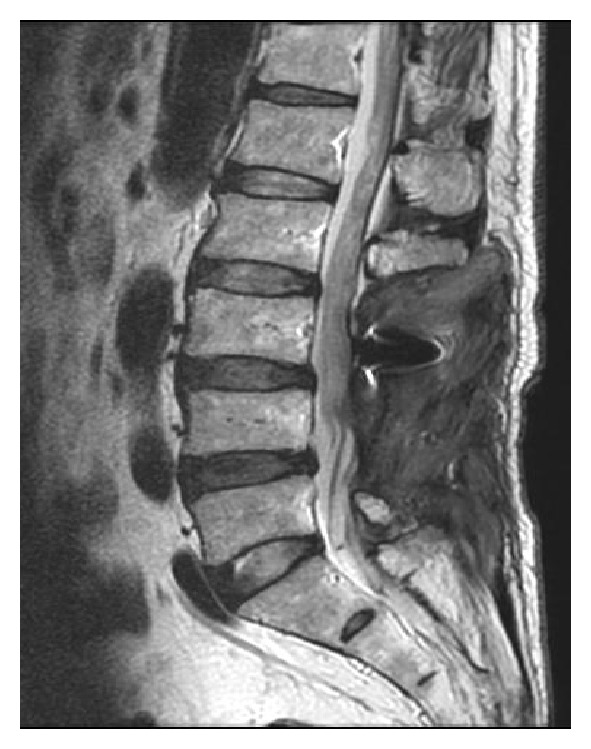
MRI T2-weighed sagittal image after hematoma evacuation shows no compression of the thecal sac.
